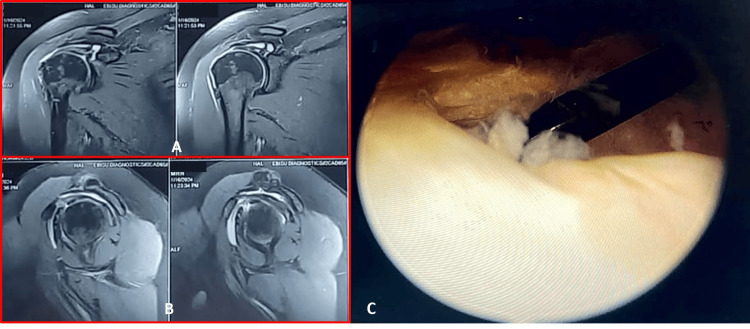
MRI and arthroscopy findings of a rotator cuff A - Coronal section MRI showing a rotator cuff tear B - Sagittal section MRI showing a supraspinatus tear C - Arthroscopic examination showing a supraspinatus tear
